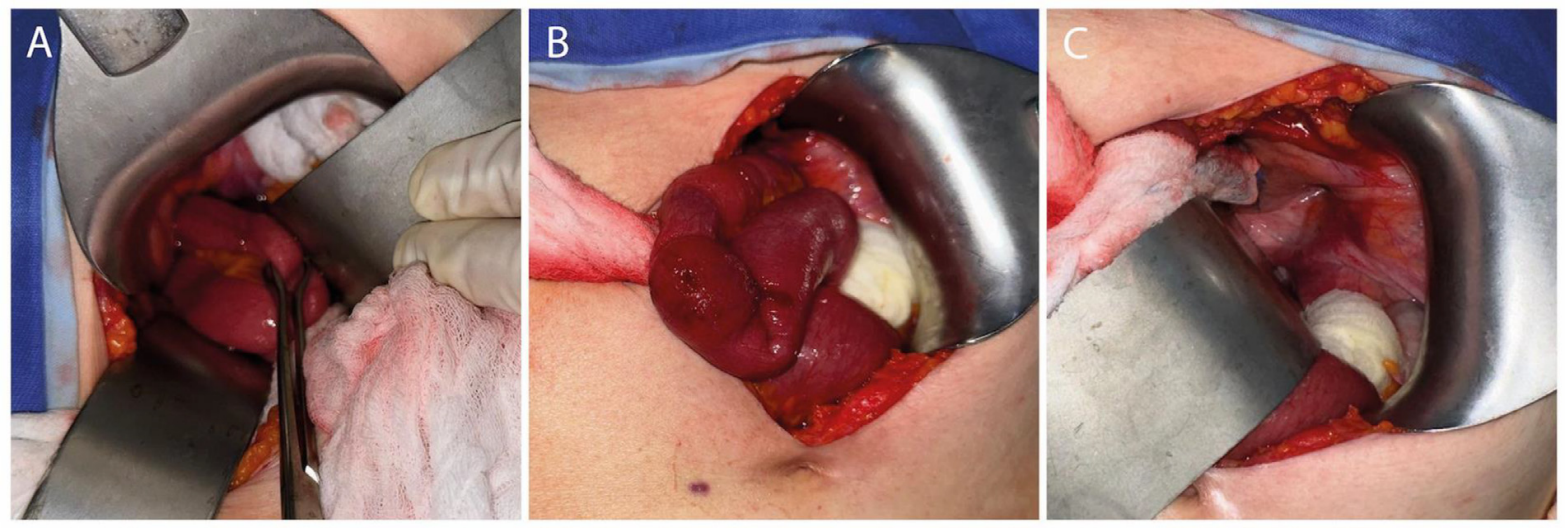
Intraoperative images showing: (A) a segment of the small bowel protruding through the right obturator foramen; (B) congestive appearance of a distal small bowel loop after hernia reduction; (C) the right obturator foramen after hernia reduction. CT, computed tomography.
